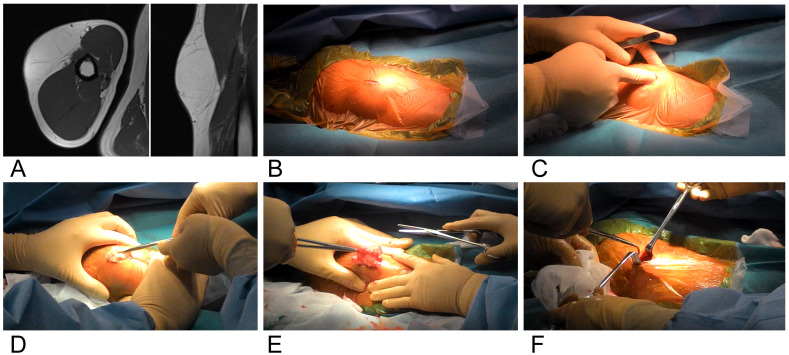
A 33-year-old male with a lipoma of the upper arm. The MRI shows a lesion with a high-intensity signal on T1-weighted images, and the signal pattern is similar to subcutaneous adipose tissue. A: Retaining ligaments are demonstrated as lines with low-intensity signal. B: In the operation, a one-inch incision is made at the center of the lipoma. C: Detachment of the lipoma from the retaining ligaments is performed bluntly with a finger. D and E: The released lipoma is extracted in a piecemeal fashion or by using a “squeeze technique”. F: Compote resection can be confirmed visually through the incision given the skin laxity. Residual lipomas can be easily removed with forceps.
